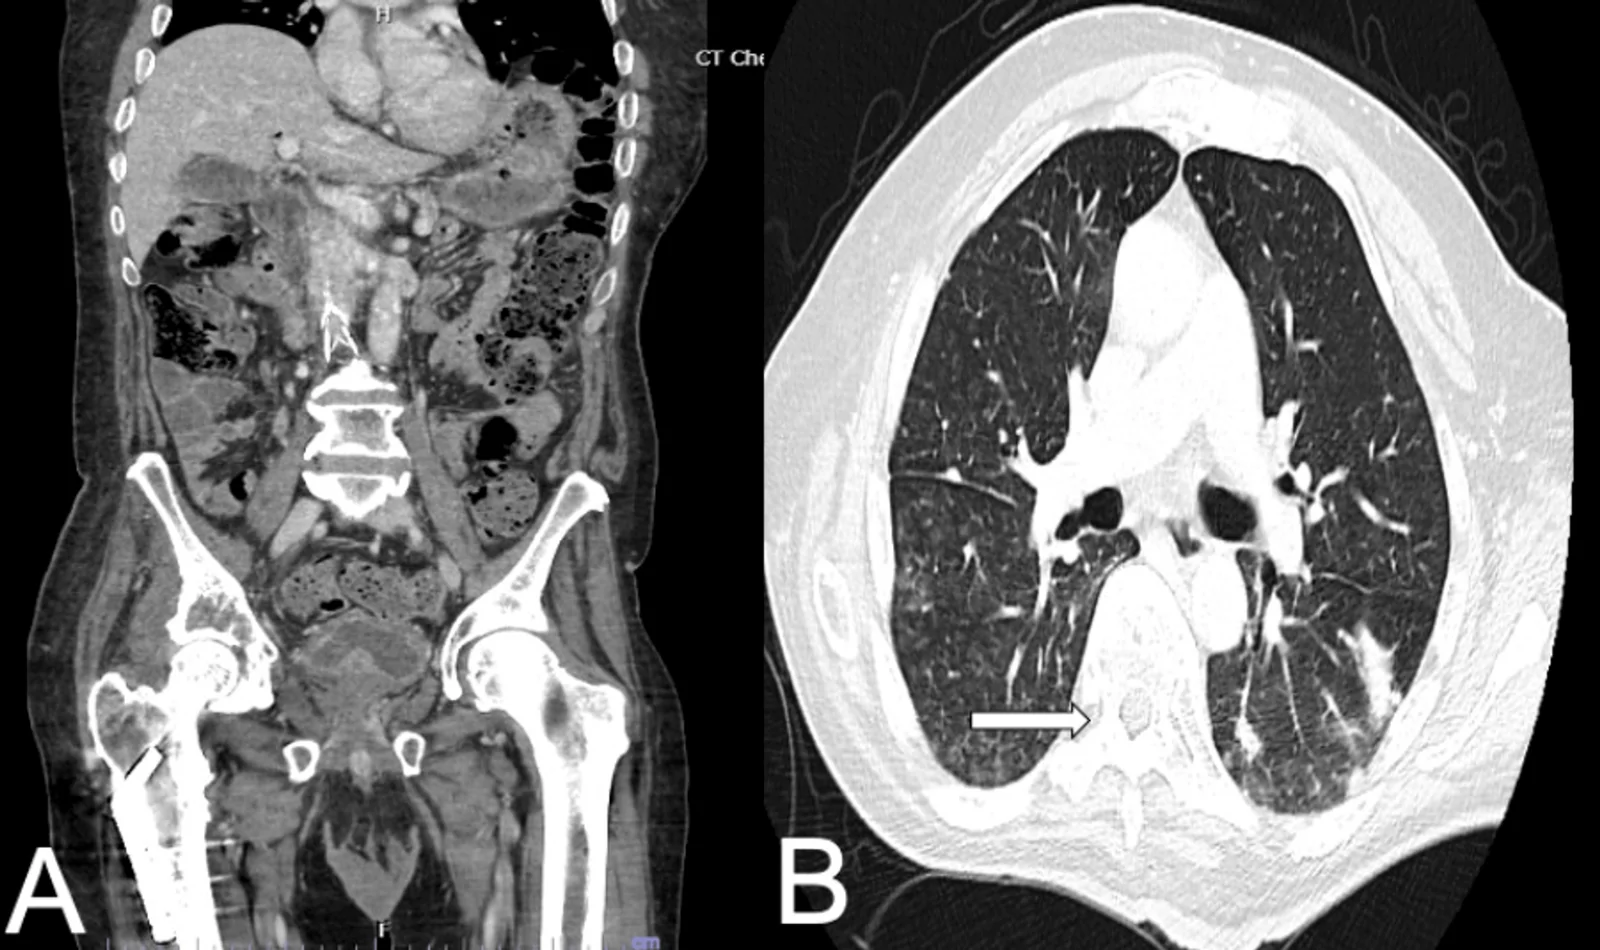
CT chest/abdomen/pelvis with intravenous (IV) contrast from the August 2025 admission A: Coronal slice of CT of the chest, abdomen, and pelvis; B: Axial slice of CT of the chest, with the white arrow indicating thoracic spinal cord compression.
